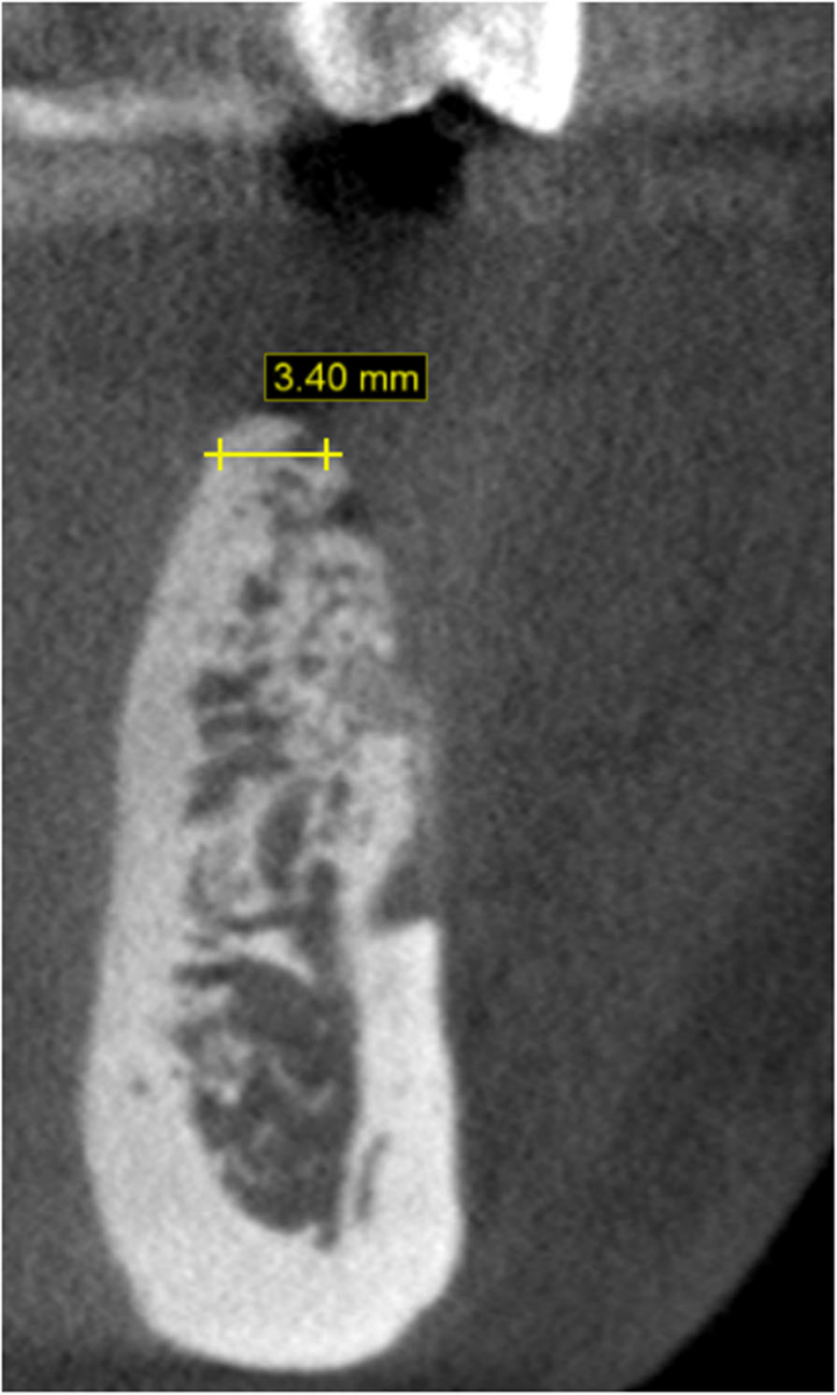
Baseline CBCT demonstrating horizontal ridge deficiency
